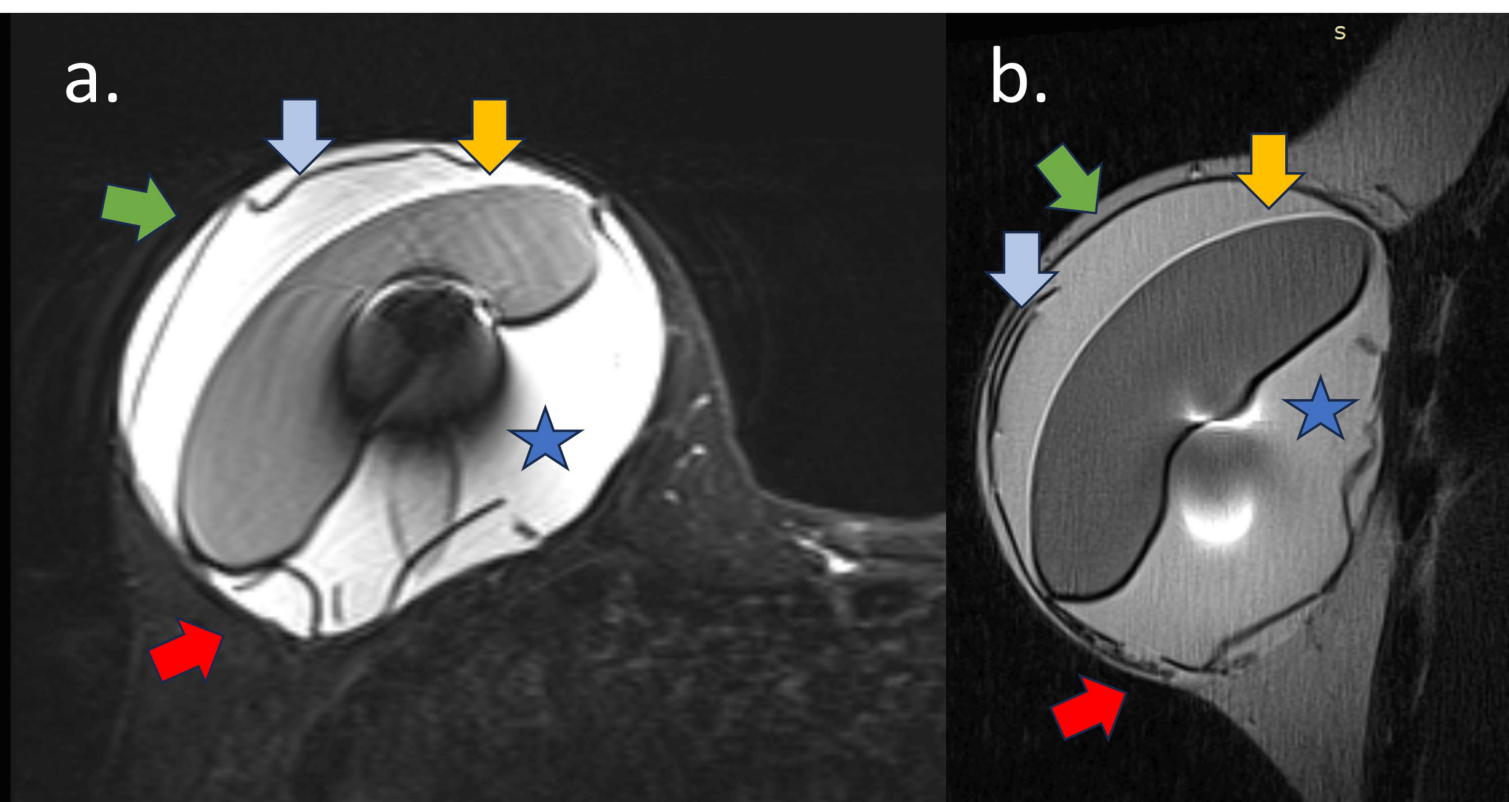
Early complications of ADM on MRI A 44-year-old woman with a retroglandular implant and ADM for six months. (a) Axial STIR-weighted sequence and (b) sagittal PD-weighted sequence. Voluminous intracapsular collection (blue star) with fibrous capsule irregularity (green arrow), implant surface (yellow arrow), intracapsular heterogeneous tissue compatible with ADM (blue arrow), and intracapsular vegetation (red arrow)
